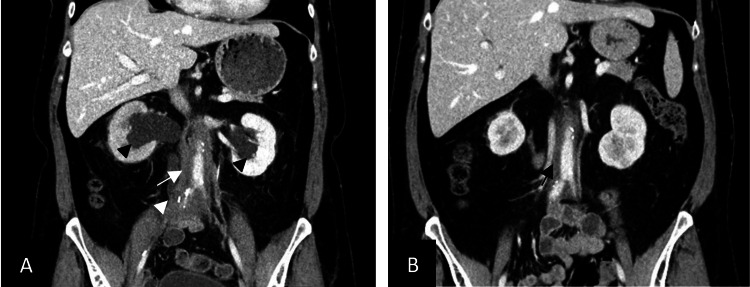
Coronal CT scan slices depicting evolution of aortitis to fibrosis (A) (white arrow) and regression of fibrosis after corticosteroid therapy (B) (black arrow). Also note the right ureteritis (white arrowhead) and bilateral hydronephrosis (black arrowheads).
